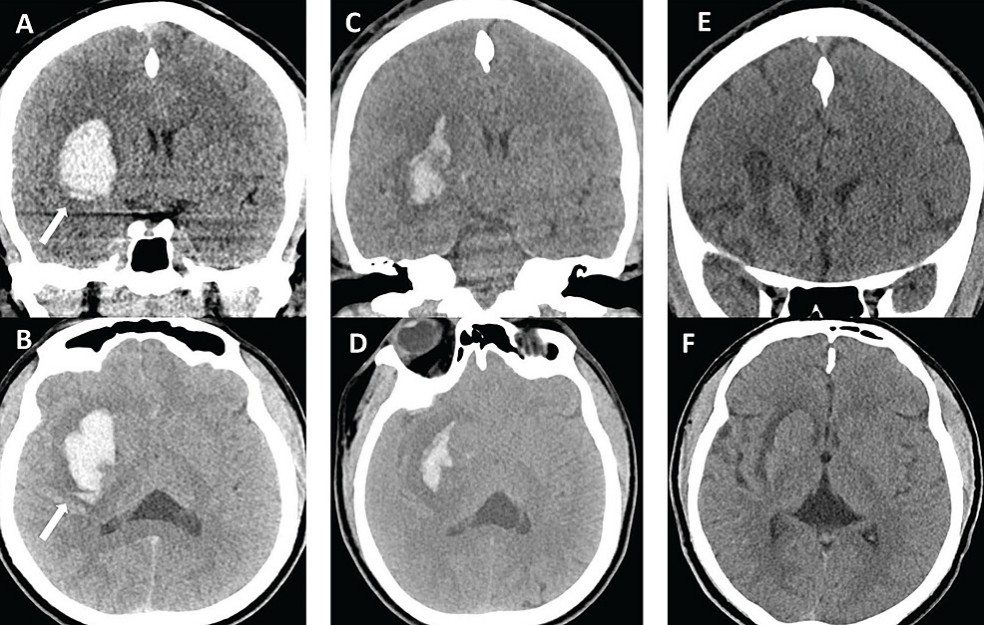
Case 1 - initial admission pre- and postprocedure and follow-up imaging. Preprocedure initial admission non-contrast coronal (A) and axial (B) head computed tomography (CT) demonstrates a 21.4 mL right basal ganglia hemorrhage (arrow). Postprocedure coronal (C) and axial (D) non-contrast CT demonstrated 44.4% evacuation with remaining hematoma measuring 11.9 mL. Coronal (E) and axial (F) follow-up imaging three months post hemorrhage shows resolution.
